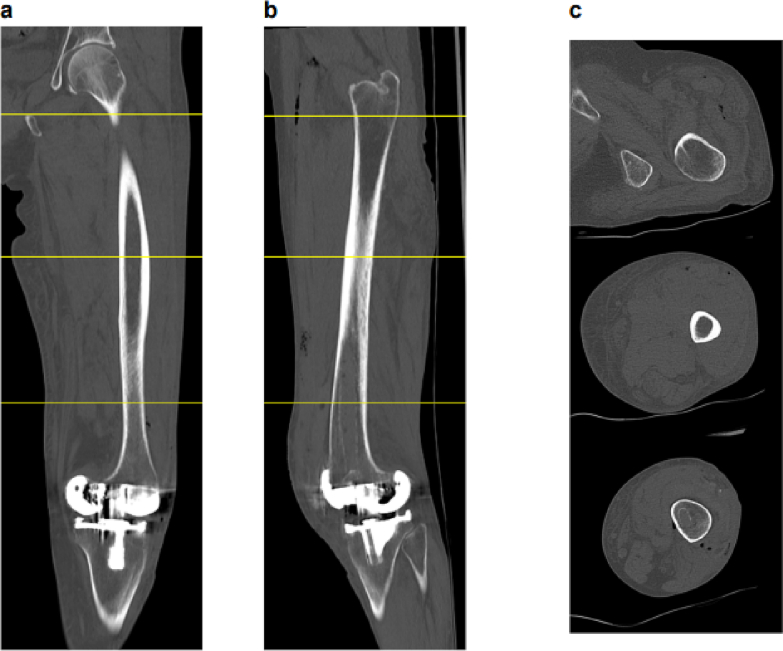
A CT scan was performed on the fifth day after the operation. a–c) Overall, the emphysema is resolving.
